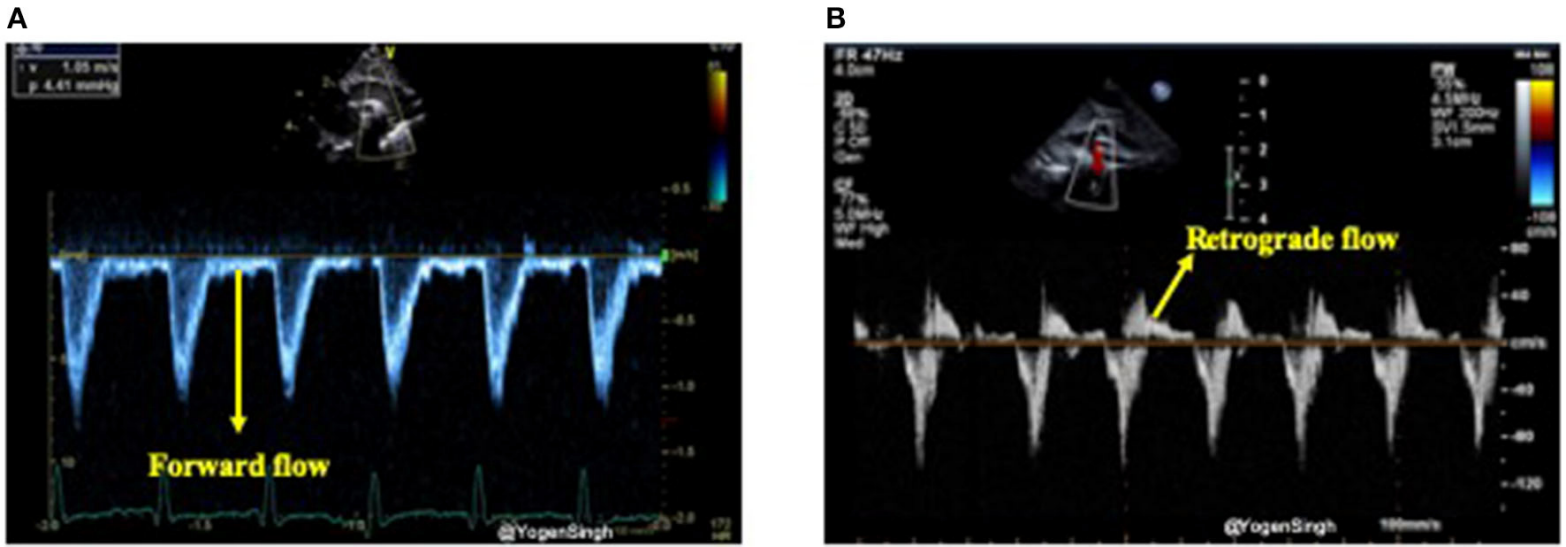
Doppler assessment of blood flow in descending aorta (post-ductal flow). (A) Showing forward blood flow during disatole; and (B) showing retrograde blood flow during diastole indicating “ductal steal” in presence of a large PDA.
